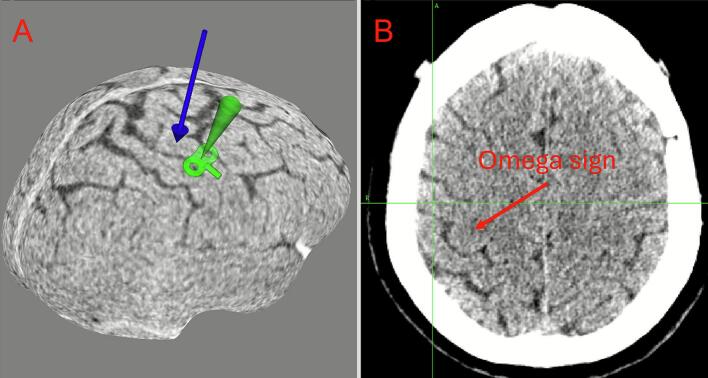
Case 1 neuronavigation target. A. Thin‑slice CT 3D reconstruction with target overlay near the inferior precentral gyrus. The blue arrow marks the M1 hand area; the green shading denotes the stimulation field of the figure‑of‑eight coil, targeting the facial motor area. B. An axial image of the stimulation plane. (For interpretation of the references to colour in this figure legend, the reader is referred to the web version of this article.)
